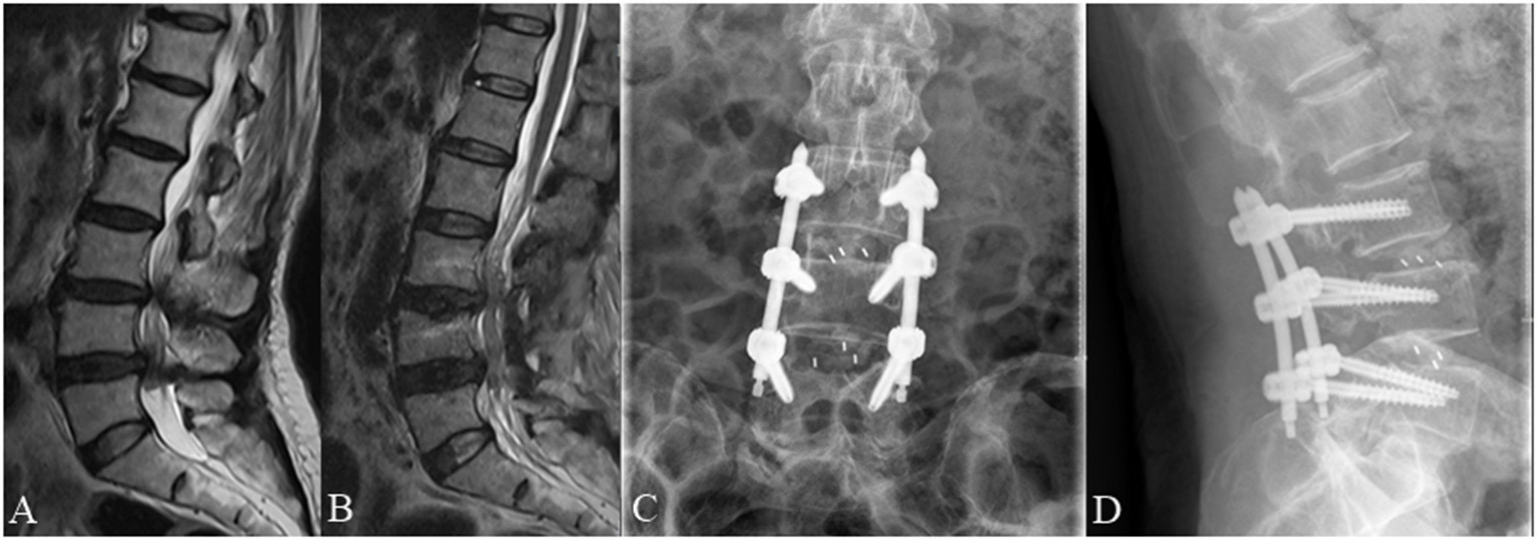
A 70 year old female complained of low back pain and right lower extremity pain for 2 months before she underwent posterior lumbar interbody fusion at L3/4 and L4/L5. (A) Pre-operative lumbar MRI showed that the nerve roots were redundant between the L3/4 and L4/L5 intervertebral disc. (B) Post-operative MRI obtained 3 days after surgery showed significant improvement with resolution of RNRs. (C,D) Lumbar radiographs obtained 3 months after surgery demonstrate internal fixation in good position without cage subsidence or migration.
